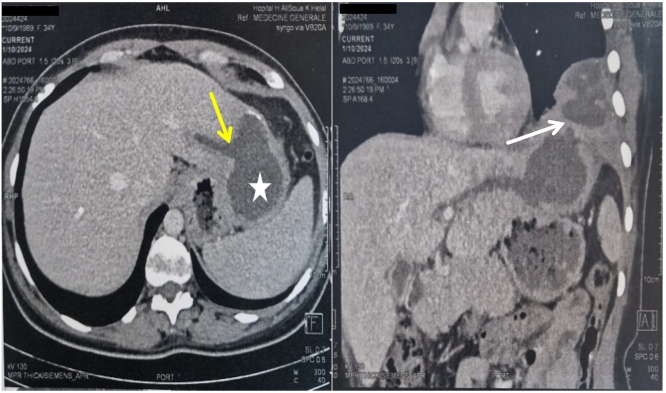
Thoraco-abdominal CT scan showing a 60 × 82 mm hydatid cyst in segment II of the liver (star) that communicated with the dilated left intrahepatic bile ducts via a 6 mm fistula (yellow arrow) and a large bronchial fistula(white arrow). (For interpretation of the references to colour in this figure legend, the reader is referred to the web version of this article.)●Preoperative consultation with a thoracic surgeon was conducted. The surgeon concluded that thoracic involvement was minimal and thoracotomy was not required.Following adequate preparation, she underwent a left subcostal surgical approach. Surgical exploration identified a 70 mm hydatid cyst in segment II, causing displacement of the lesser gastric curvature and establishing a communication with the left hemithorax through a 40 mm diaphragmatic breach. Importantly, no additional phrenectomy was required, as the fistula was successfully managed through the existing diaphragmatic defect (Fig.2).
